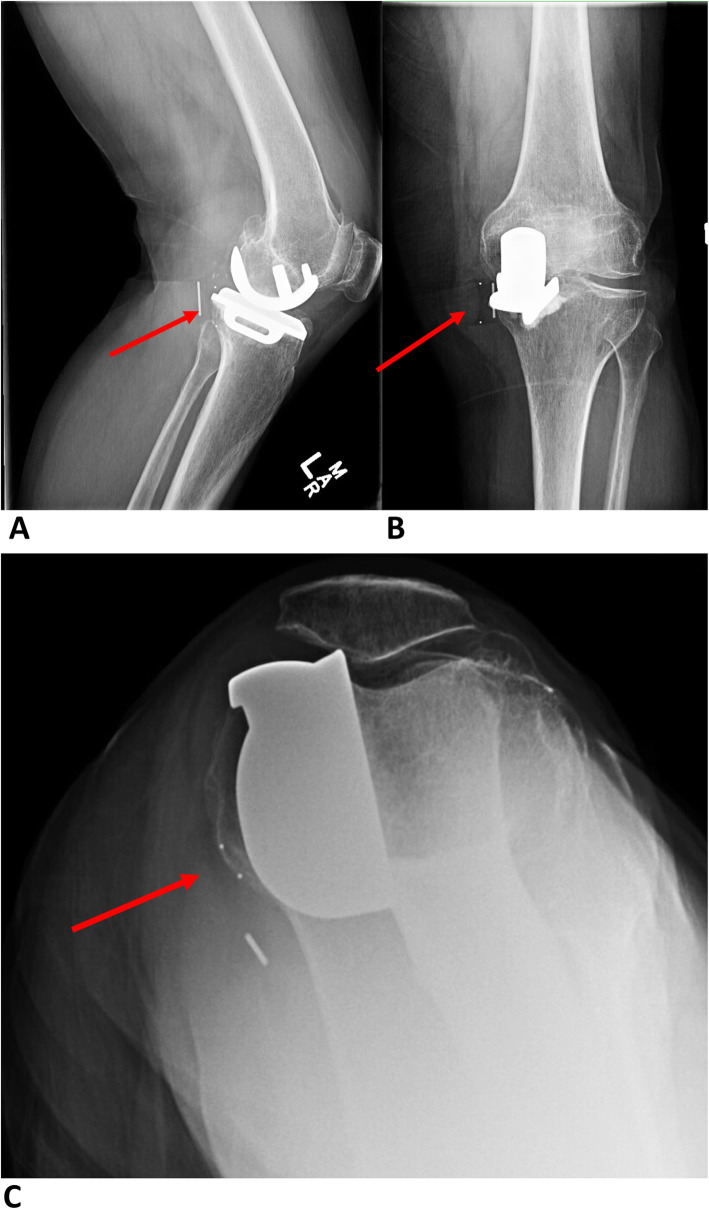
(a) Lateral, (b) AP, and (c) sunrise radiographs of the left knee demonstrating a cemented medial Oxford unicompartmental knee arthroplasty with posteromedial dislocation of the polyethylene mobile bearing insert. There is also significant lateral and patellofemoral osteoarthritis with associated anterior femoral and patellar osteophytosis
